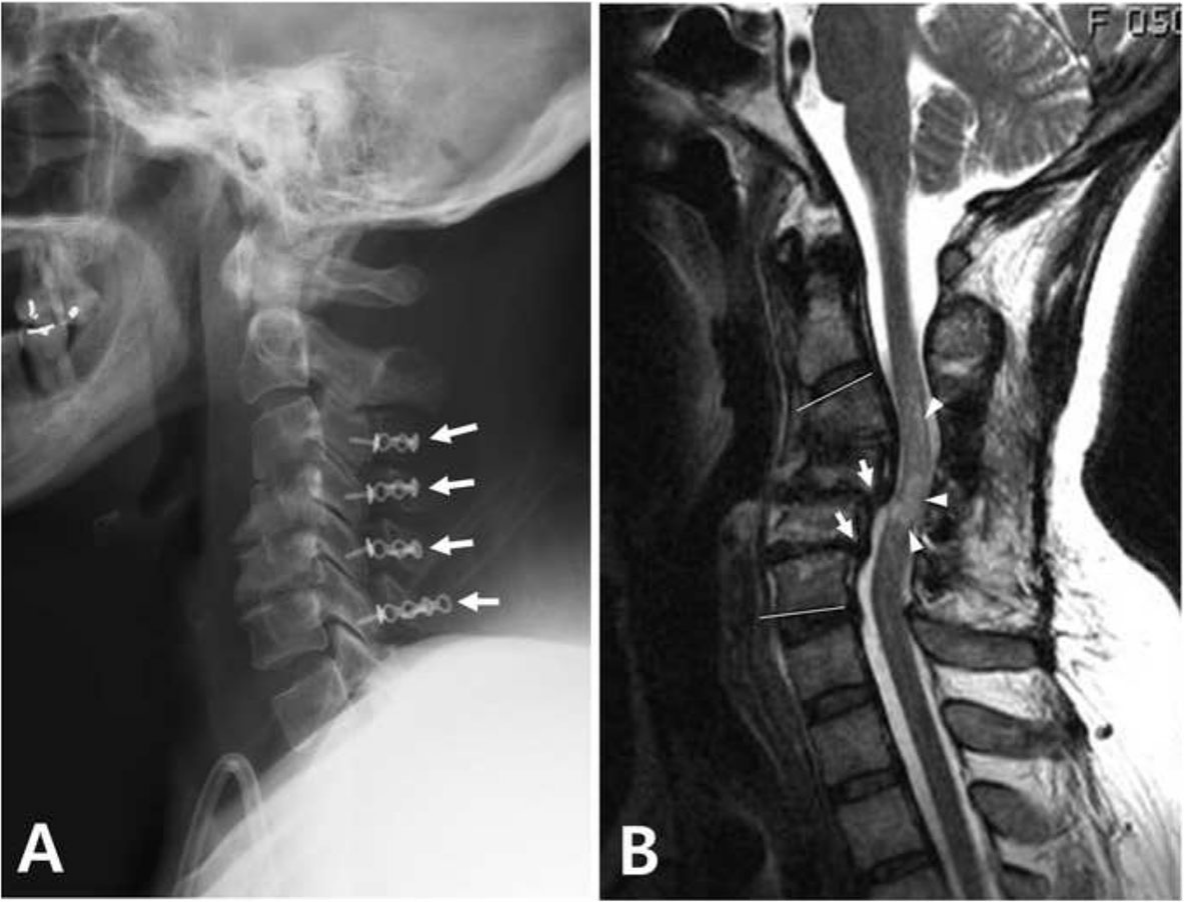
Postoperative images. a Immediate postoperative lateral radiograph of the cervical spine shows the laminoplasty state from C3 to C6 using plates and screws for fixation (*arrows*). b T2-weighted sagittal magnetic resonance image obtained 8 months postoperatively shows retrolisthesis (*arrows*), kyphosis, and a signal change in the spinal cord (*arrow heads*) at C3-C5. Complete regression of the epidural abscess is noted
